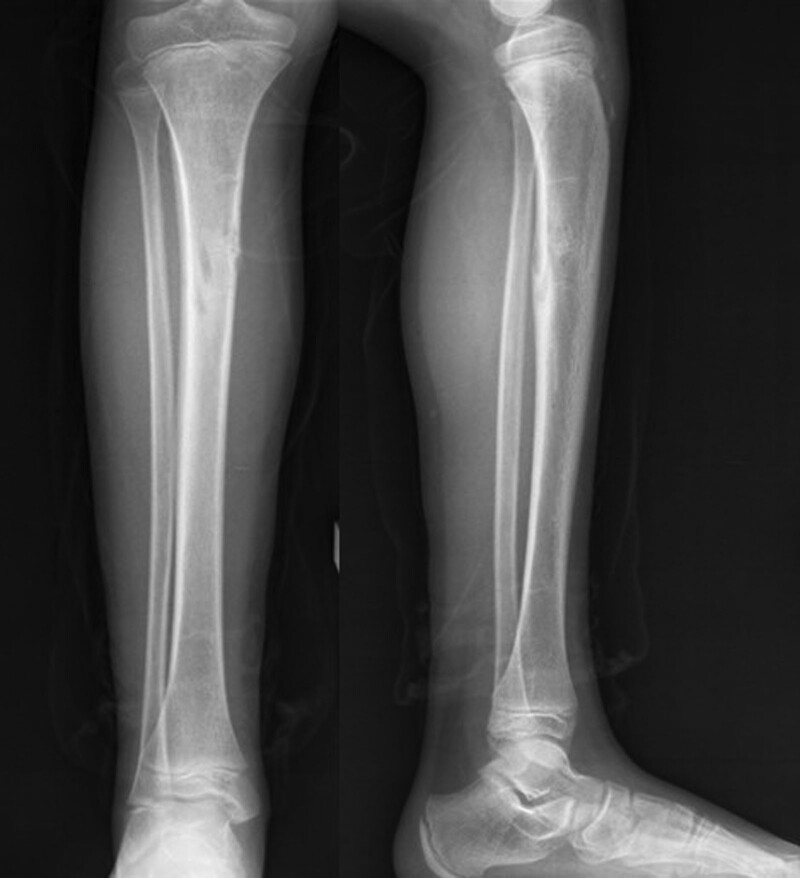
This patient (number 2) was followed up for 8 yr. Final cruris radiographs show partial ossification of the lesions.
